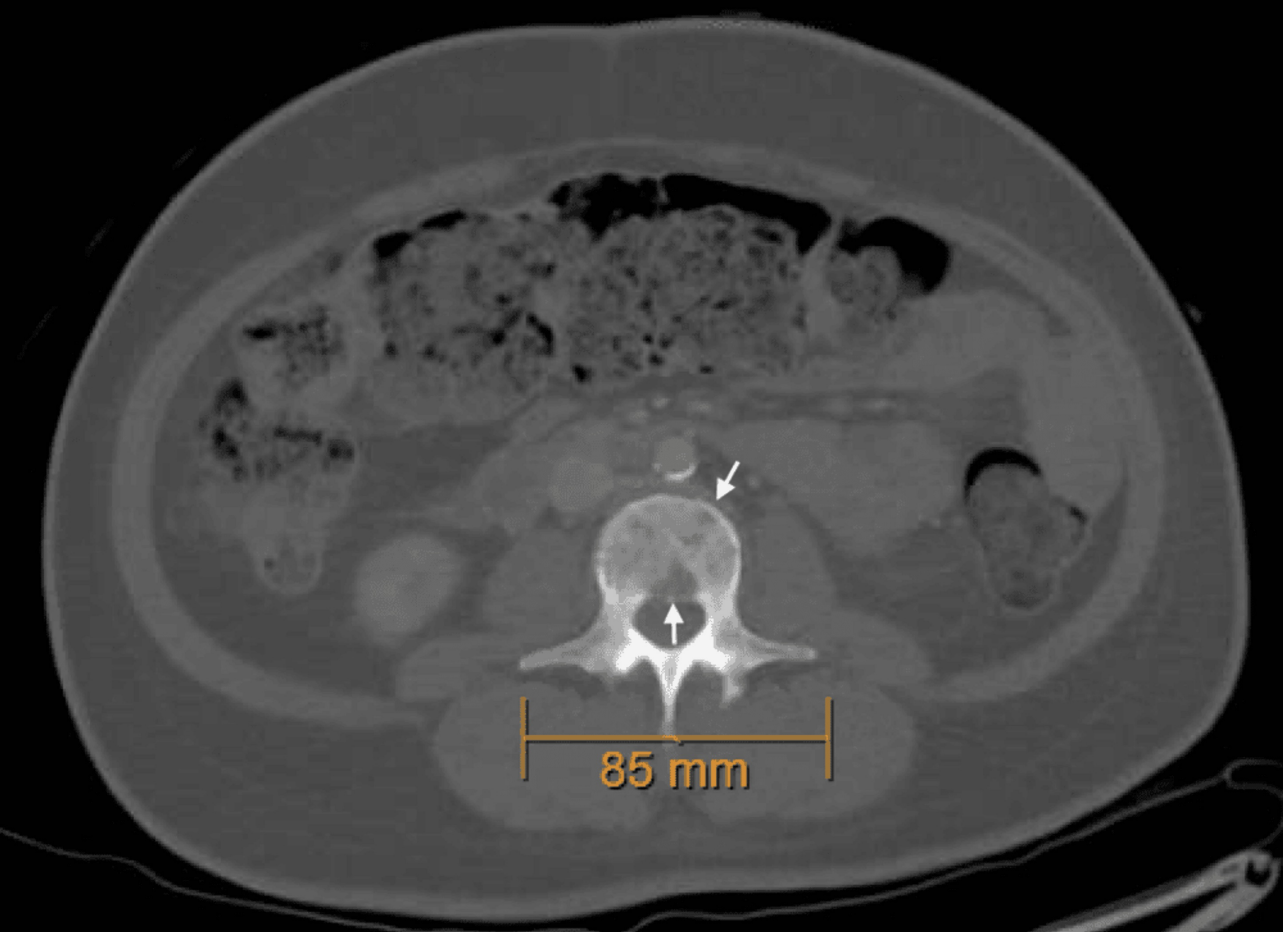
CT scan of the abdomen and pelvis (axial view) demonstrating innumerable lytic lesions on the vertebral body (white arrows)
